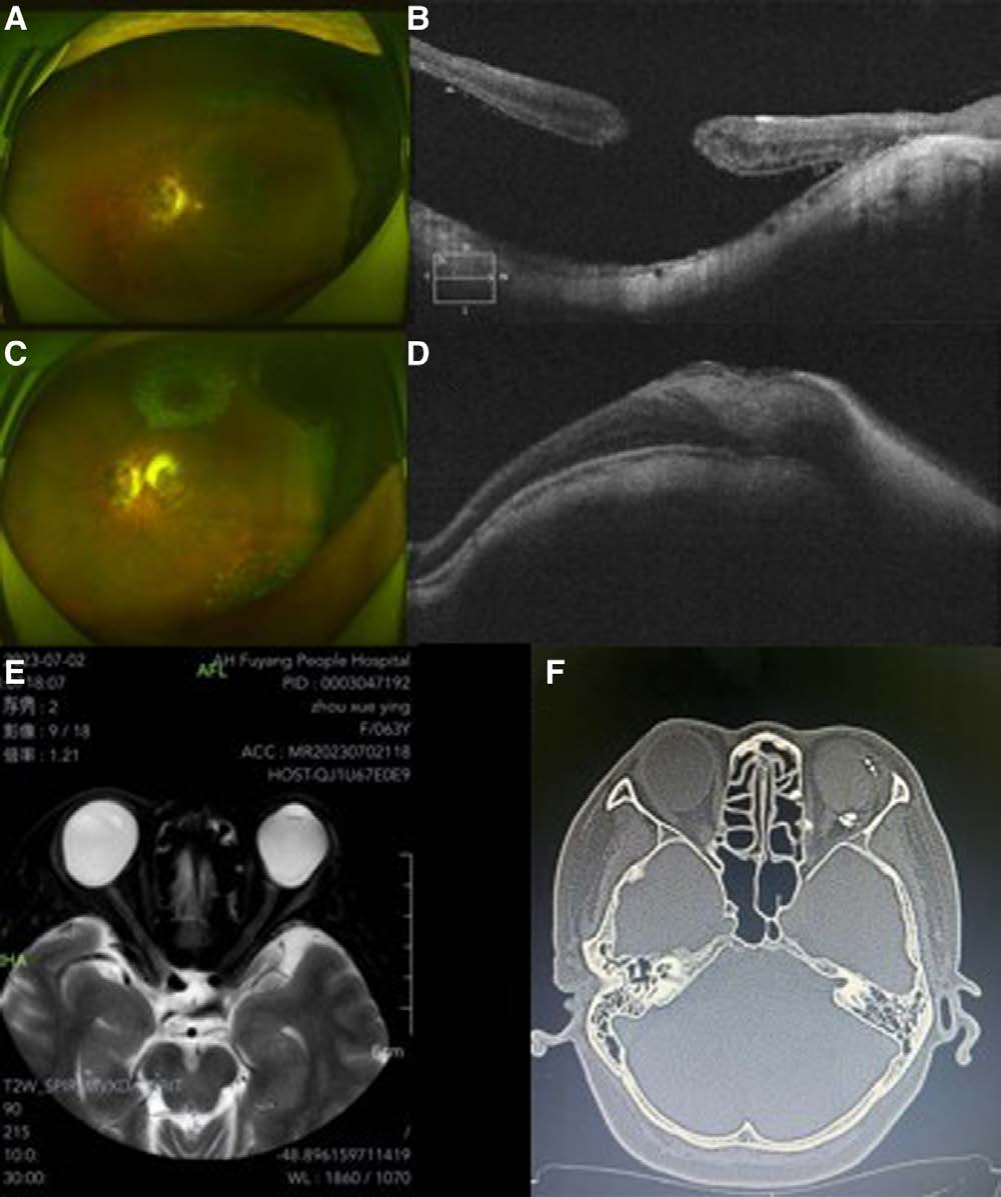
Pre- and postoperative fundus photography, OCT images, and orbital imaging of a 63-year-old woman’s left eyes. Ocular examination demonstrated retinal detachment encompassing the posterior pole with a macular hole and staphyloma (A, B). Magnetic resonance imaging (MRI) showed significant posterior bulging of the sclera (E). One week postoperative examination showed reattachment of the retina with macular hole closure (C, D). The macular buckling explant was stable and positioned away from the optic nerve (F). OCT = optical coherence tomography.
